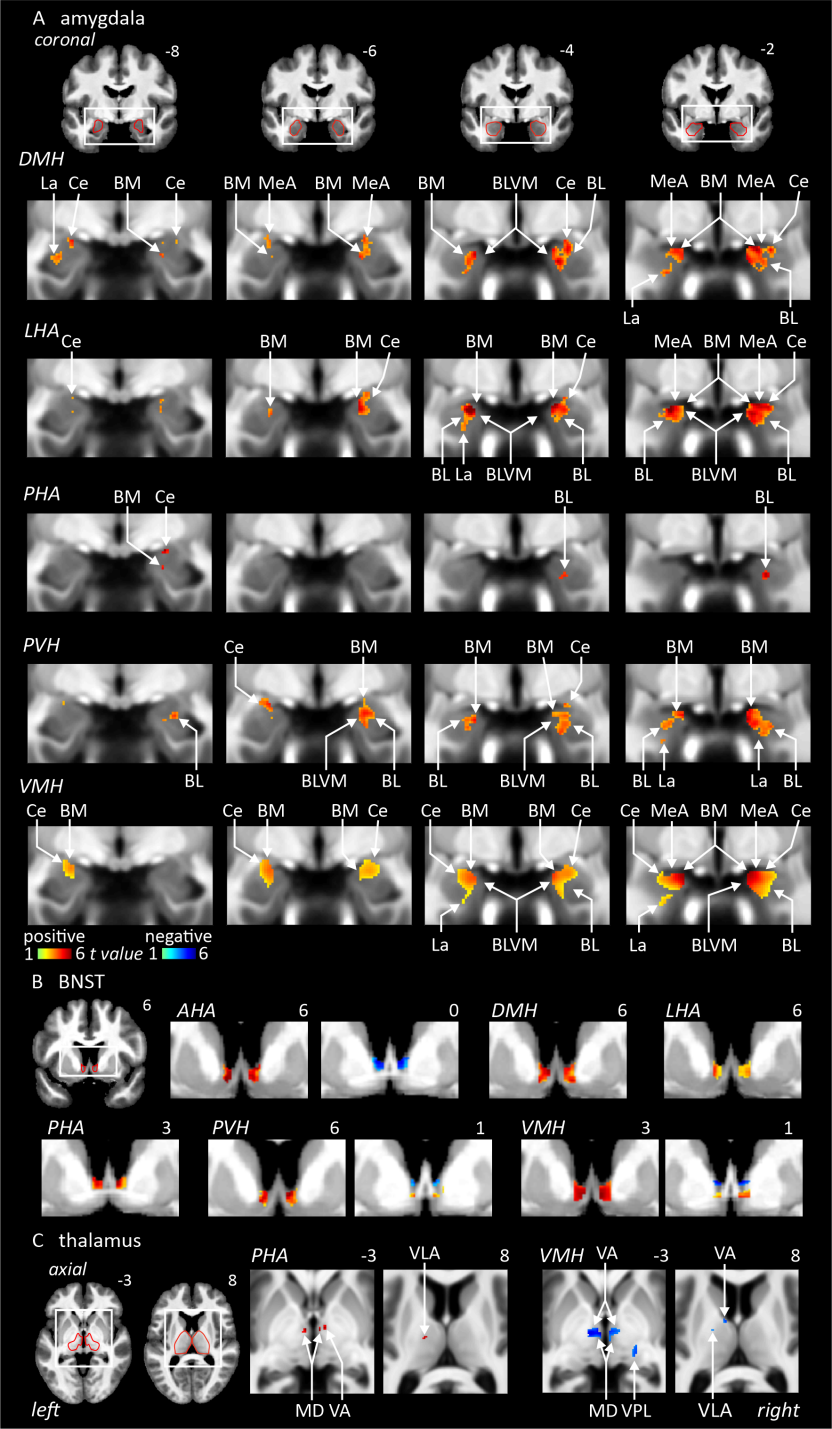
Resting connectivity between six hypothalamic seed regions and the amygdala, bed nucleus of the stria terminalis, and thalamus (n = 150). Areas of significant positive (hot colour scale) and negative (cool colour scale) connectivity overlaid onto a series of coronal and axial mean T1-weighted anatomical images. Slice locations in Montreal Neurological Institute Space are indicated at the top right of each slice. Red outlines indicating region of interest masked applied for the analysis. (A) Amygdala. (B) Bed nucleus of the stria terminalis. (C) Thalamus. For abbreviations, see table.
